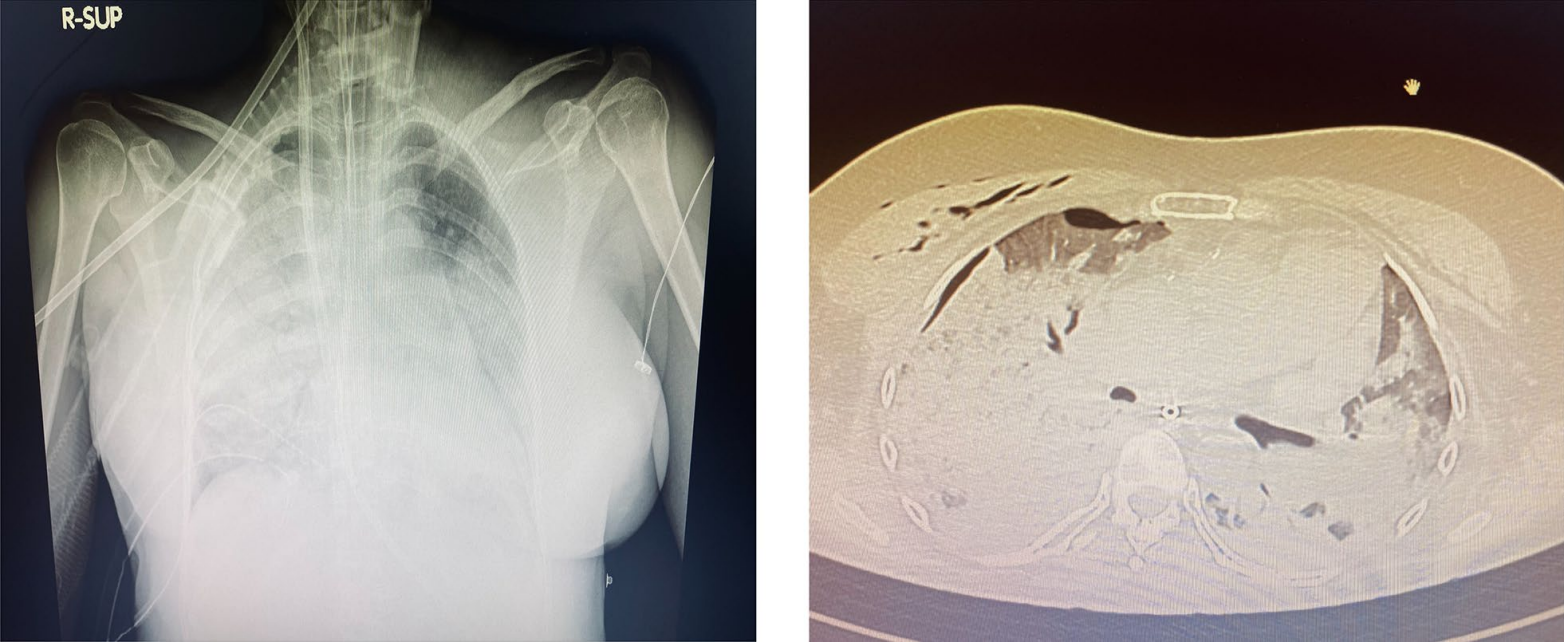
The chest X-ray and computerized axial tomography before the ECMO and surgical procedure
